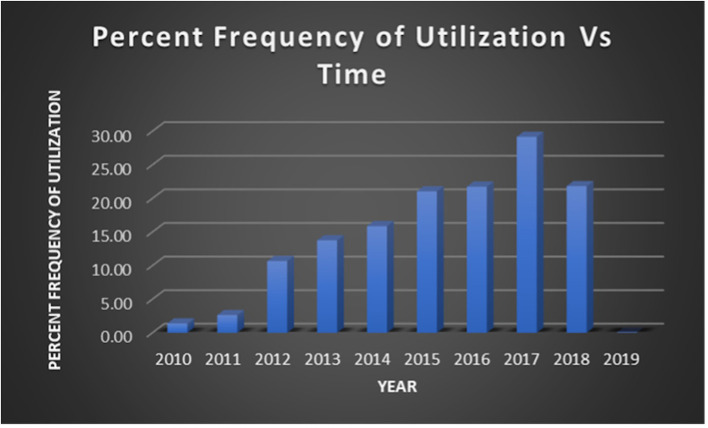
Utilization of post-DCL CT over time from January 2010 to February 2019.
